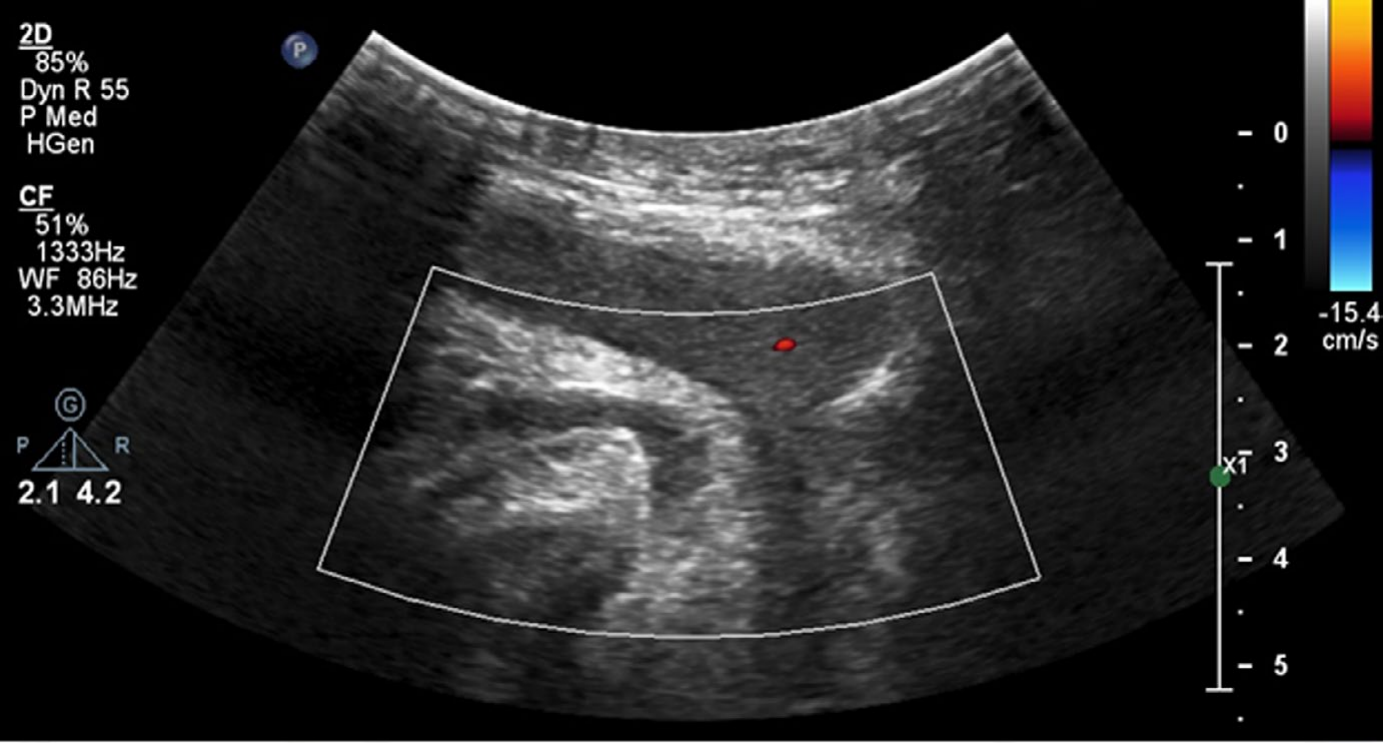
Ultrasound evaluation of the deep dorsal penile vein (Arrow) shows no flow in the vein.star: corpus spongiosum
